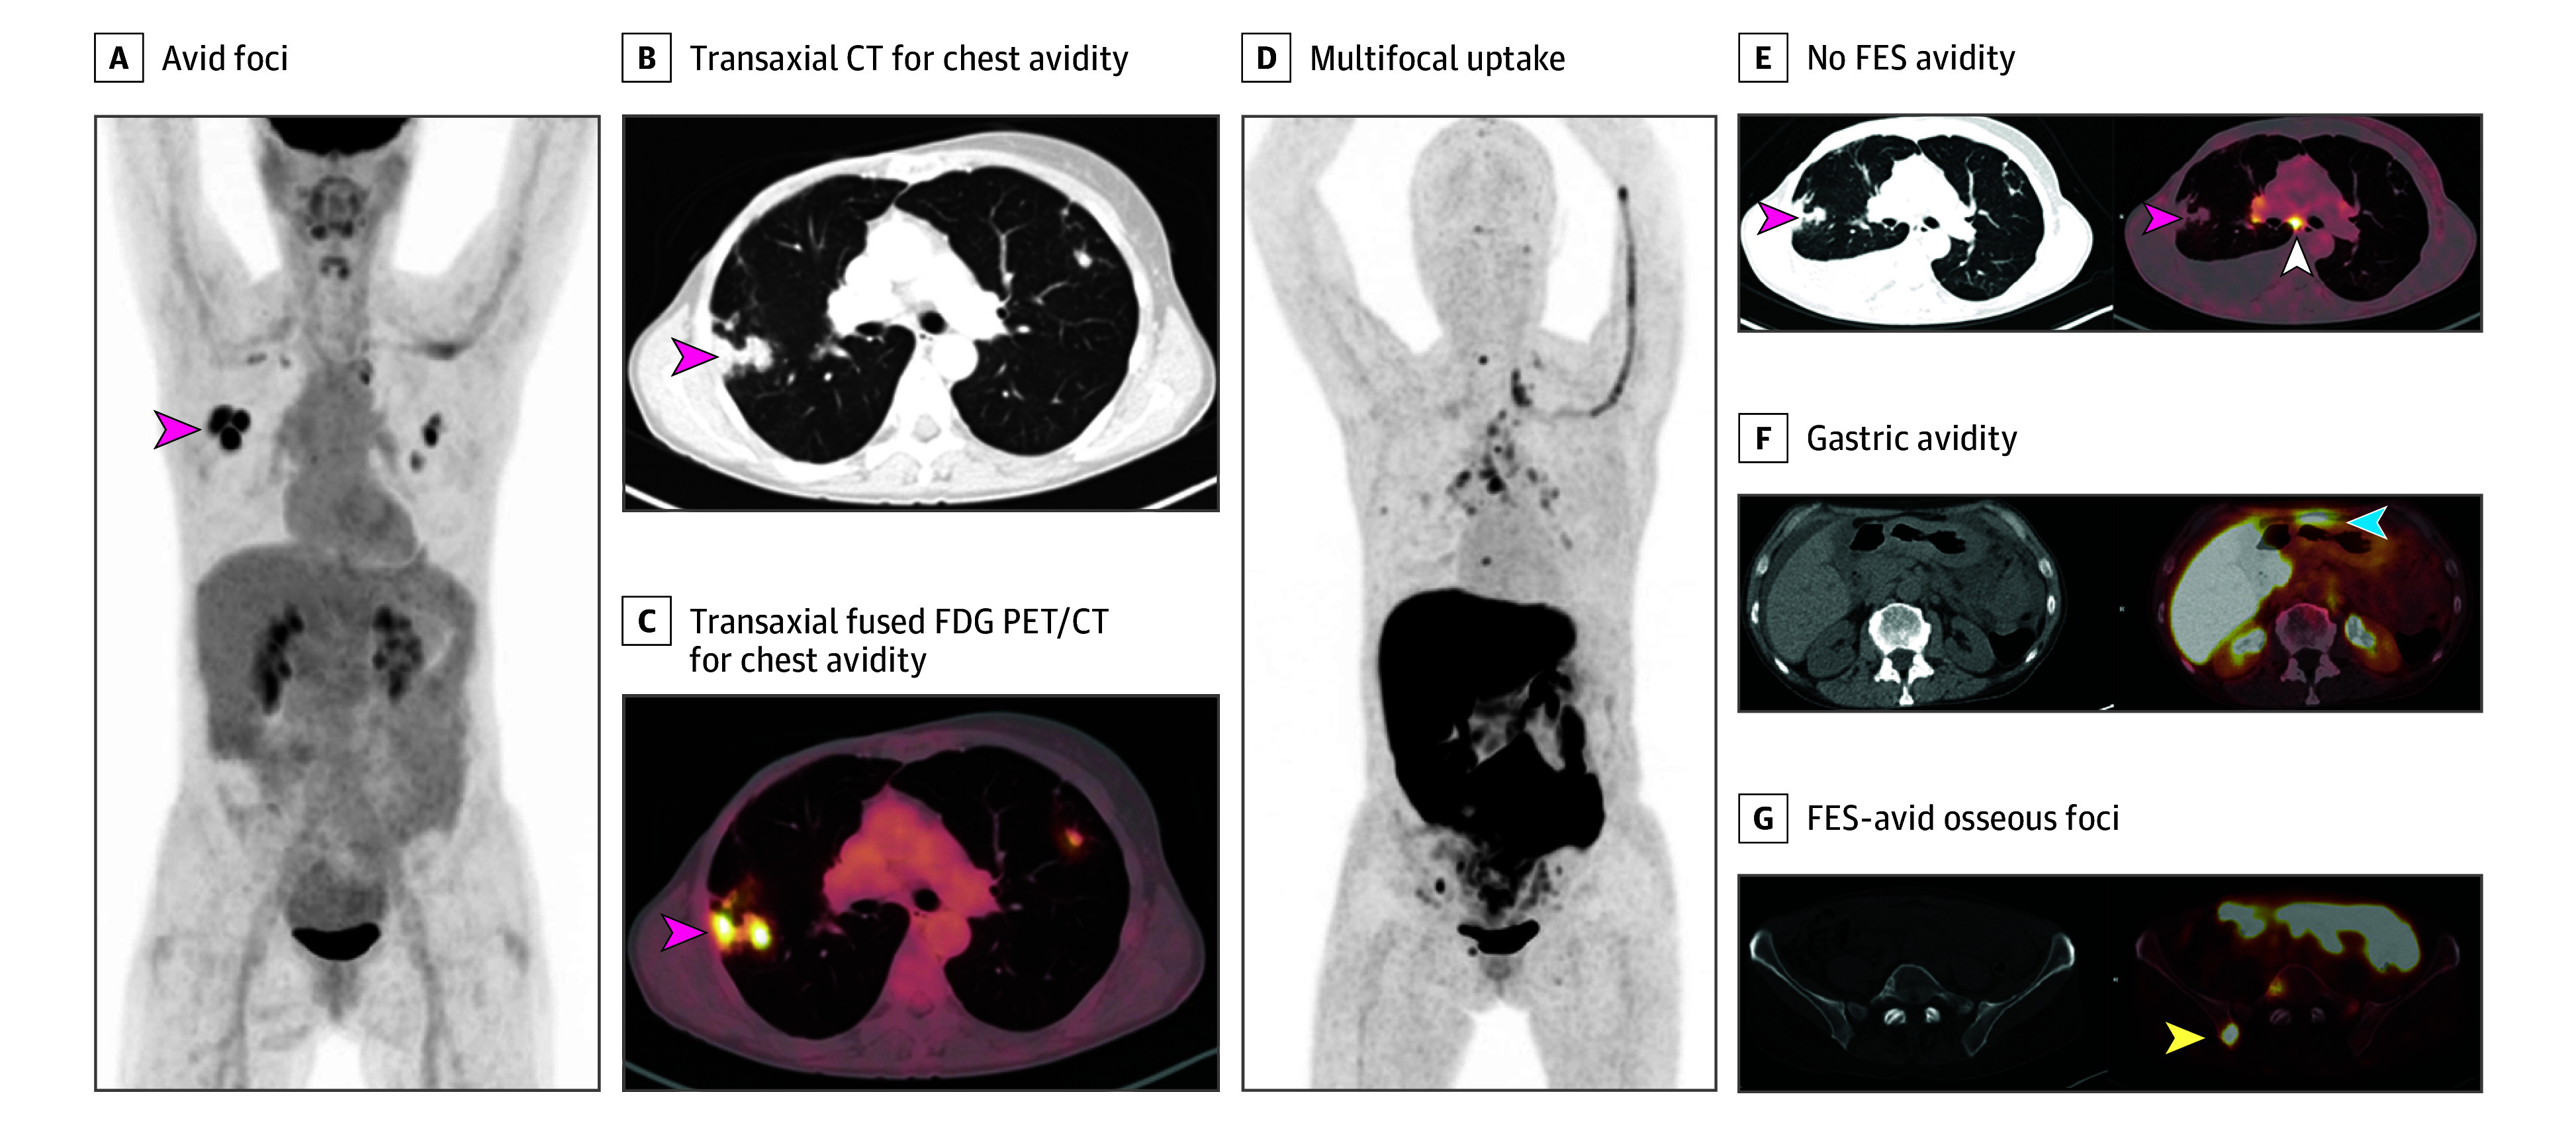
Recurrent Disease Detected on 16α-^18^F-fluoro-17β-estradiol (FES) Position Emission Tomograph (PET) Computed Tomography (CT) but Not Detected on ^18^F-fluorodeoxyglucose (FDG) PET/CT Patient was a woman in her 50s with suspected recurrence of invasive lobular breast cancer. A, Maximum intensity projection image from FDG PET/CT demonstrating avid foci in the chest (red arrow). Transaxial CT (B) and transaxial fused FDG PET/CT image (C) showing that the chest avidity corresponds to FDG-avid lung nodules (red arrows) suspicious for malignancy. The lung nodule was subsequently biopsied but found to represent benign granulomatous inflammation and thus a false positive on FDG PET/CT. D, Maximum intensity projection image from FES PET/CT demonstrating multifocal uptake suspicious for malignancy. E, Transaxial CT and fused FES PET/CT demonstrating no FES-avidity in the biopsy proven benign granulomatous lung nodules (red arrows), thus true negative on FES PET/CT. FES-avidity in nodes (white arrow) is suspicious for malignancy. F, Transaxial CT and fused FES PET/CT demonstrates gastric avidity (blue arrow) suspicious for malignancy. G, Transaxial CT and fused FES PET/CT demonstrates FES-avid osseous foci (yellow arrow) suspicious for malignancy. This osseous focus was subsequently biopsied and proved to be an osseous metastasis and thus true positive on FES PET/CT.
